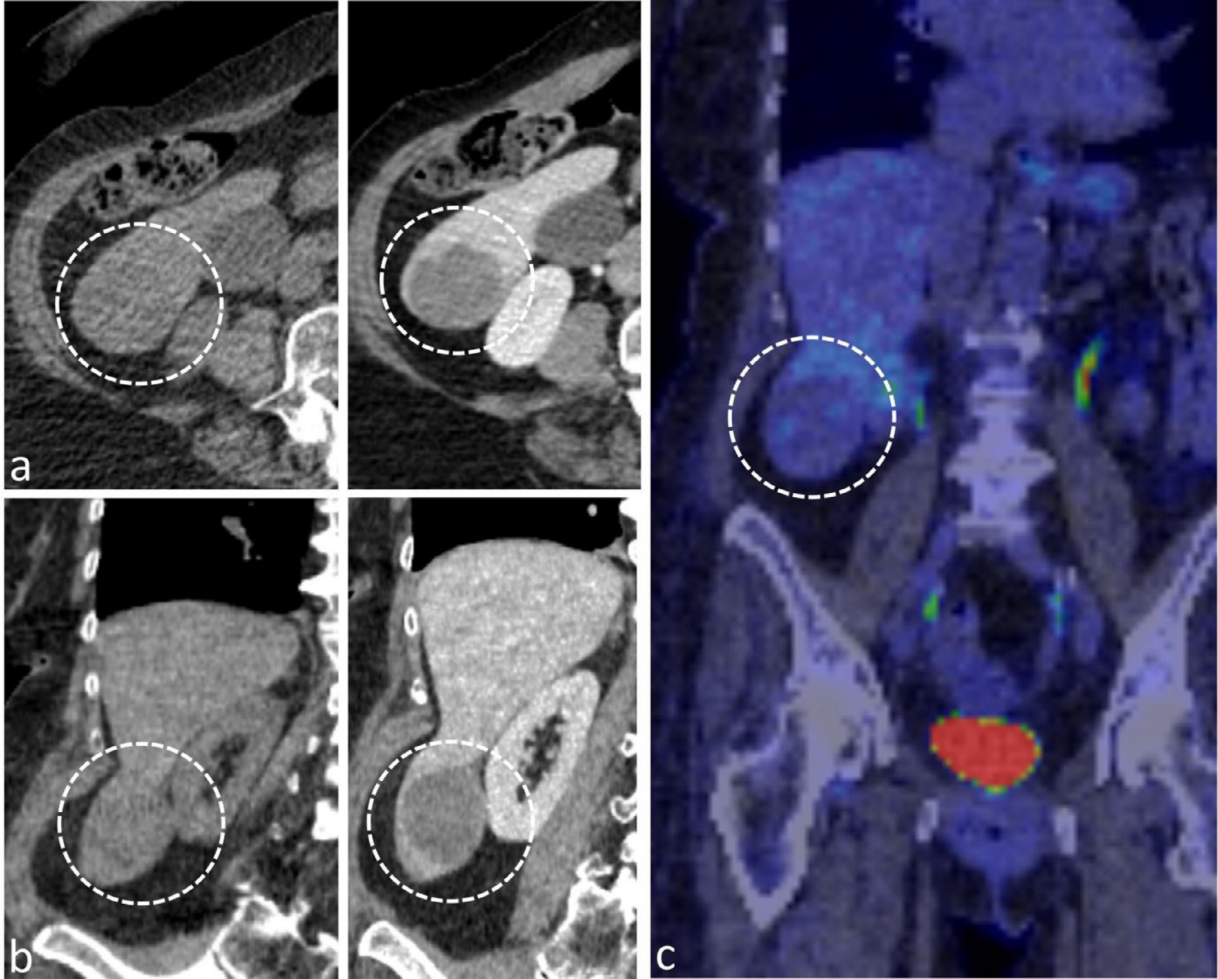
Contrast-enhanced CT and PET/CT scan. Solitary hypodense lesion without significant contrast enhancement compared to the surrounding liver parenchyma in transversal (A) and coronal plane (B) demonstrated in nonenhanced images (left) and contrast-enhanced images (right). PET/CT showed no avidity of the lesion (C).
